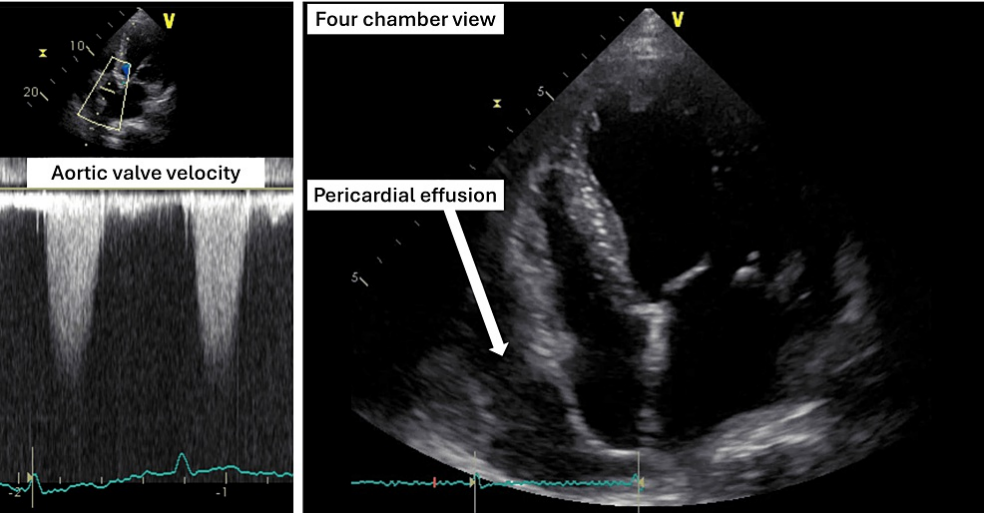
Echocardiogram showing severe aortic valve stenosis (left) and pericardial effusion (right)
